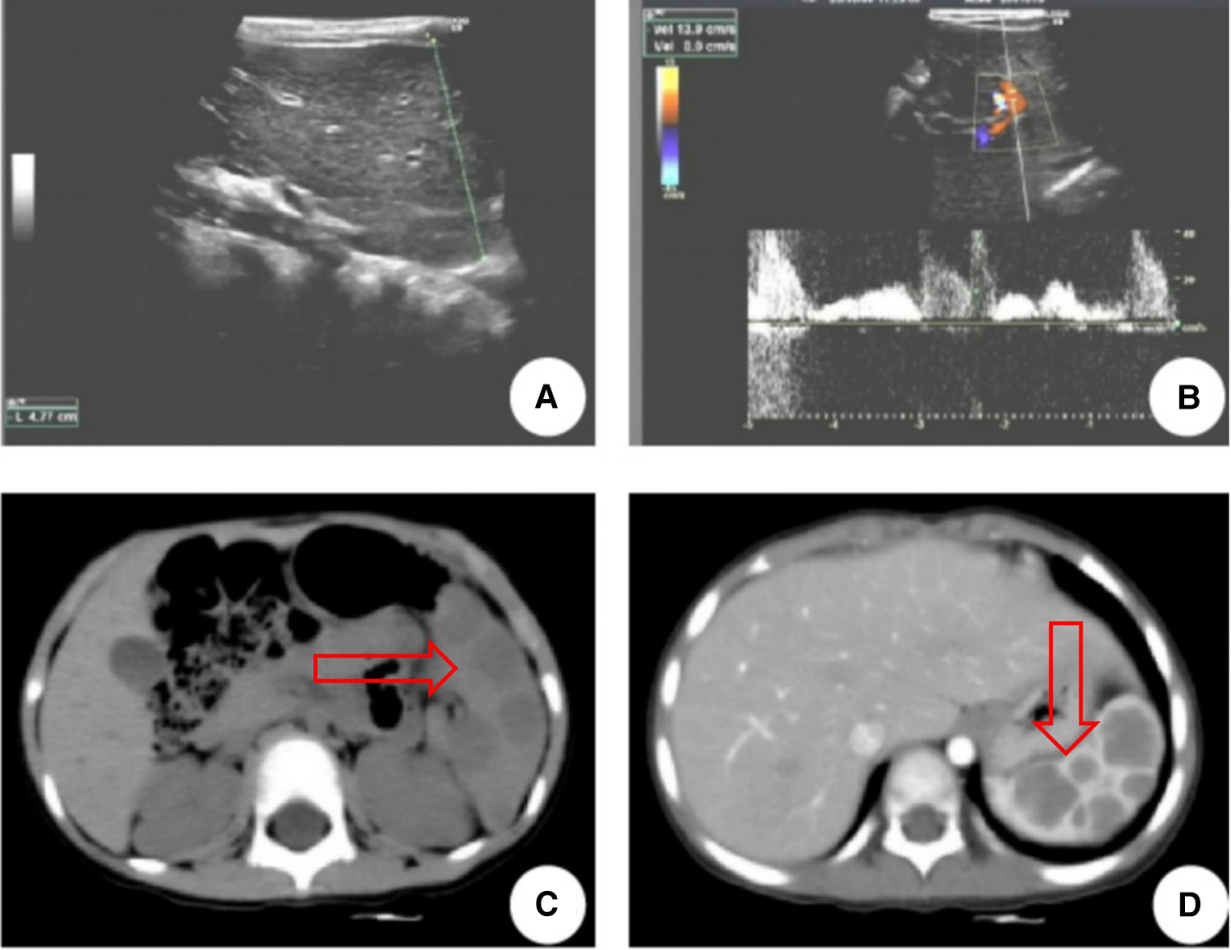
LCA patient's ultrasound and CT images. (A,B) Ultrasound indicated multiple solid slightly hyperechoic masses were diffusely distributed in the spleen, measuring approximately 26 mm × 23 mm, 12 mm × 13 mm, 19 mm × 19 mm, 9 mm × 9 mm, 8 mm × 5 mm, etc. (C) CT revealed multiple nodular lesions in the spleen. (D) Enhancement reveals multiple masses of varying sizes within the spleen, with uneven internal enhancement and weaker than the spleen. The arrows point to the tumor.
